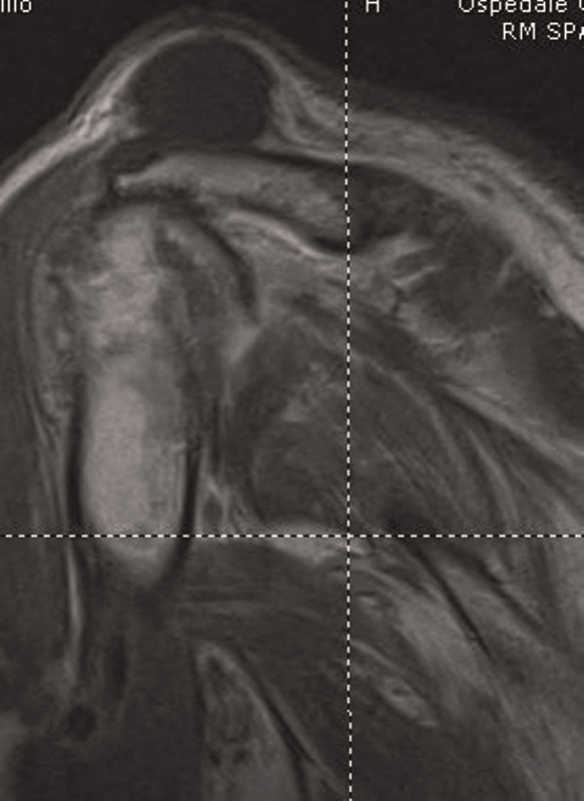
Preoperative shoulder MRI.
